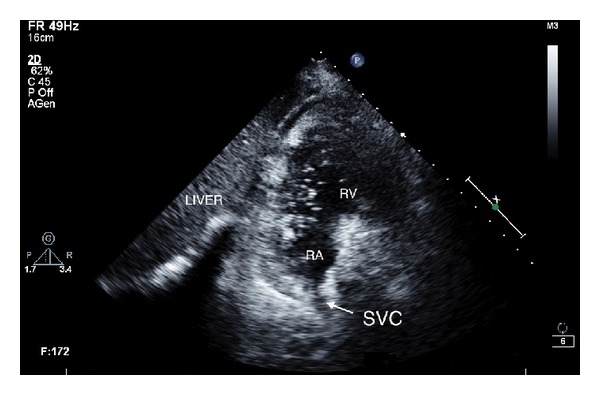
Echocardiography. Subcostal acoustic window: air bubbles in right atrial and ventricular cavities. RA: right atrium; RV: right ventricle; SVC: superior vena cava.
